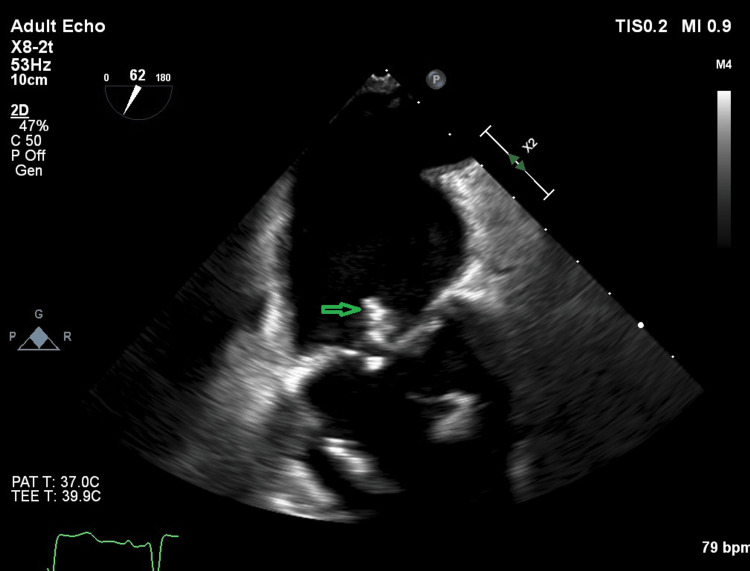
A transesophageal echocardiography of the patient with a vegetation of the mitral valve (arrow).
